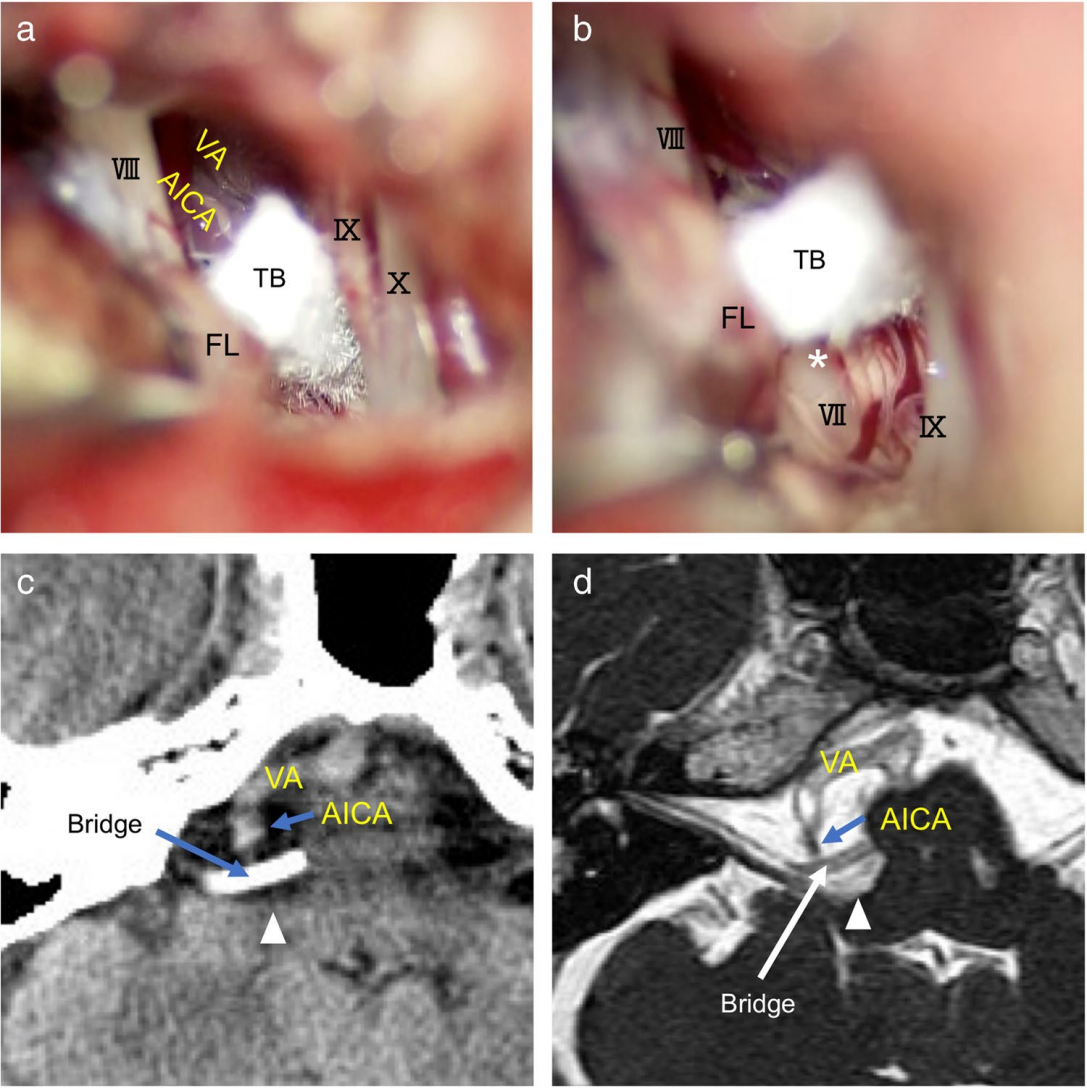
Case presentation (Case 1). A 70-year-old woman with HFS on the right caused by the VA and AICA compression. a An intraoperative photograph of MVD for hemifacial spasm on the right side. A Teflon bridge (TB) is inserted to reposition the offenders, the VA, and the AICA. The lateral edge is placed on the base of the flocculus (FL), and the other edge is placed on the brainstem near the exit of the abducens nerve. b A photo from the caudal side shows a free space over the root exit zone (REZ) of the facial nerve (white asterisk) created under the bridge. c A postoperative computed tomography (CT) taken postoperative1 week indicates the location of the bridge (white rectangular shape) and a free space over the REZ (white arrowhead). d Magnetic resonance imaging (MRI) taken 6 months after surgery shows a free space (white arrowhead) maintained in front of the REZ and the transposed offenders in place on the Teflon bridge
